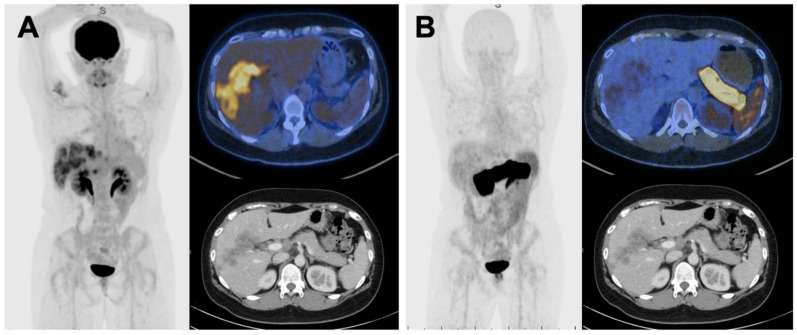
MIP and axial slices of [^18^F]FDG PET-CT (A) compared to 1 h [^64^Cu]Cu-SAR-BBN (B) in Patient 6. Diagnostic CT shows hypoenhancing liver metastases, confirmed by biopsy. These lesions were FDG positive, BBN negative.
